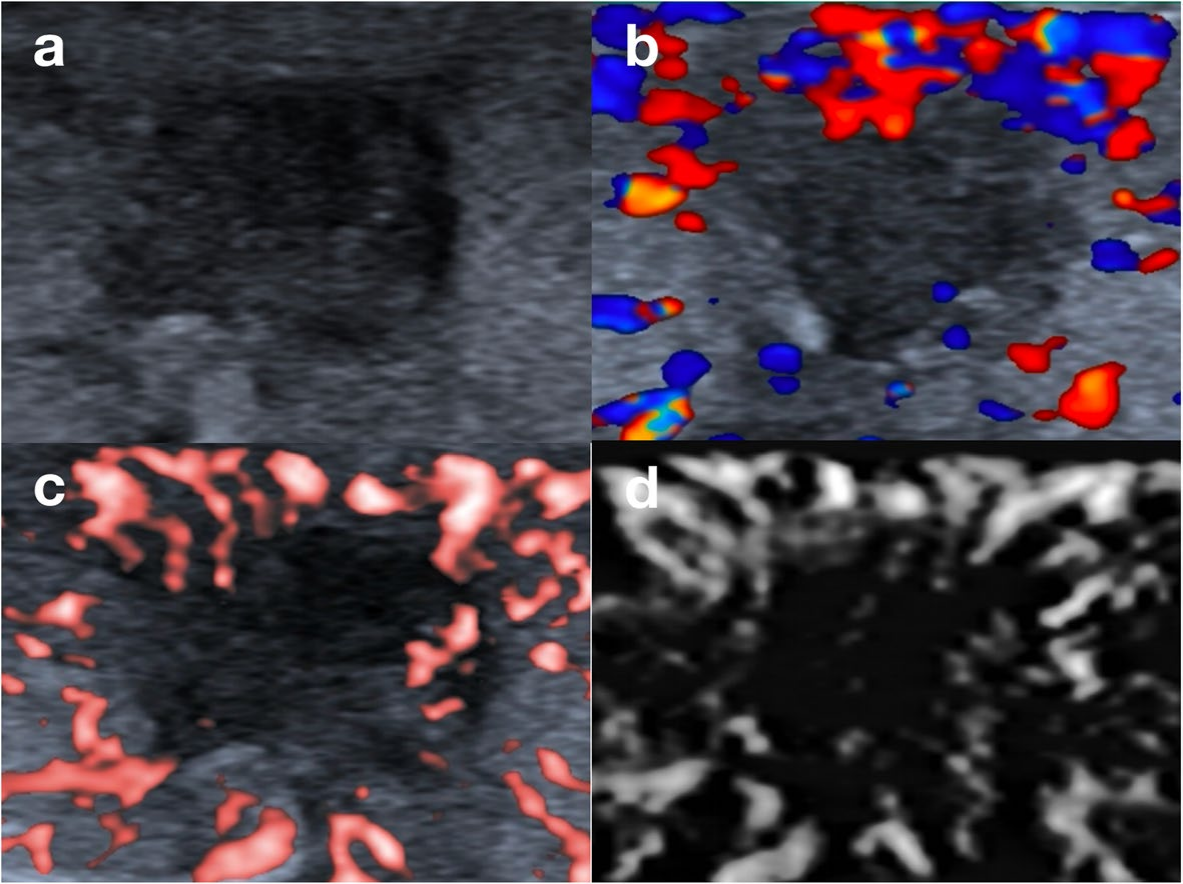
Ultrasound images of a papillary thyroid carcinoma of classic variant in a 42 female patient. a The lesion presented as a hypoechoic solid nodule with irregular margin on greyscale. b CDFI showed scattered dot- and rod-like flow signals at the margin of the nodule. c CSMI showed a crab claw-like pattern. d. MSMI showed a crab claw-like pattern
